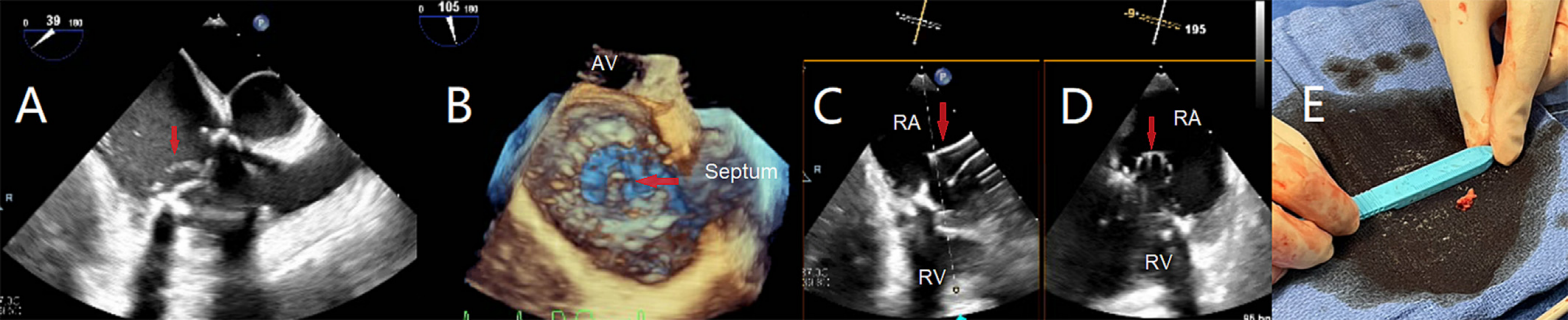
The vegetation marked by arrow on (A) two-dimensional transesophageal echocardiography (TEE); and (B) atrial perspective in three-dimensional TEE (AV, aortic valve); cannula in the right atrium viewed on X-plane after the aspiration was complete — (C) longitudinal; and (D) cross-sectional of the funnel (RA, right atrium; RV, right ventricle); (E) vegetation's gross appearance after removal.
